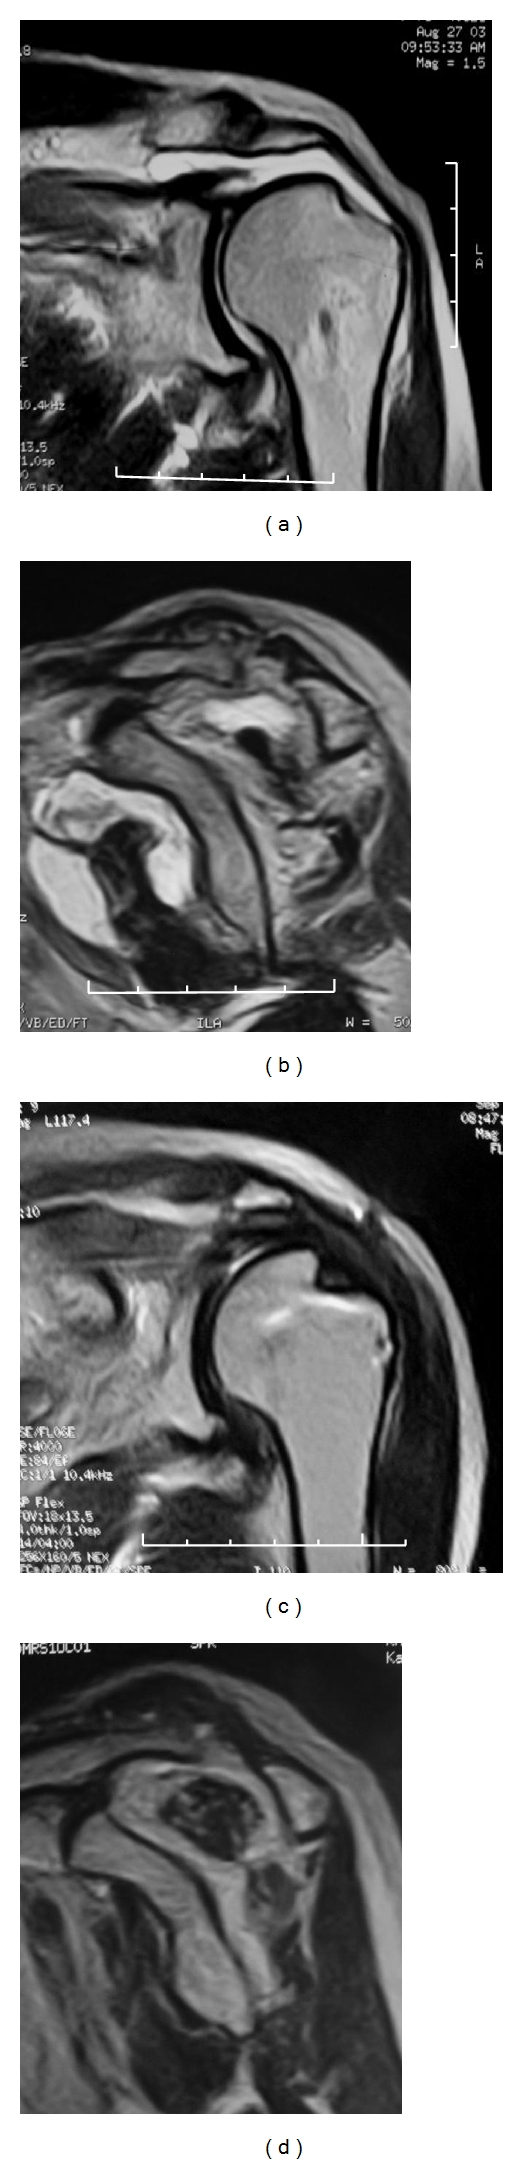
Case 1. (a) Pre-op MRI; oblique coronal, (b) oblique sagittal. (c) Post-op MRI (48 months after surgery); oblique coronal, (d) oblique sagittal.
